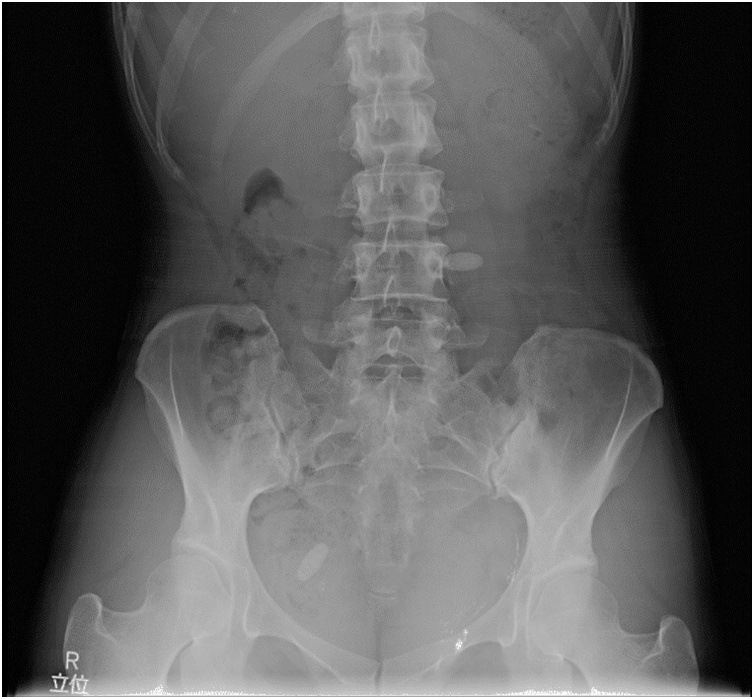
An abdominal X-ray examination after laparoscopic inguinal herniorrhaphy. No dense materials in the peritoneal cavity was recognized.
